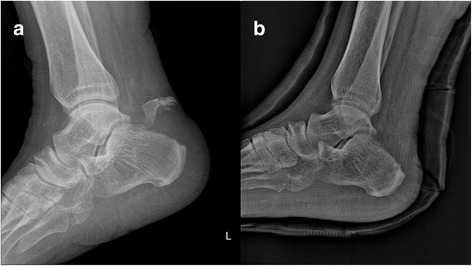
A calcaneal avulsion fracture in the preoperative X-ray (a) and the fragment attached to the Achilles tendon removed in the postoperative X-ray (b)
